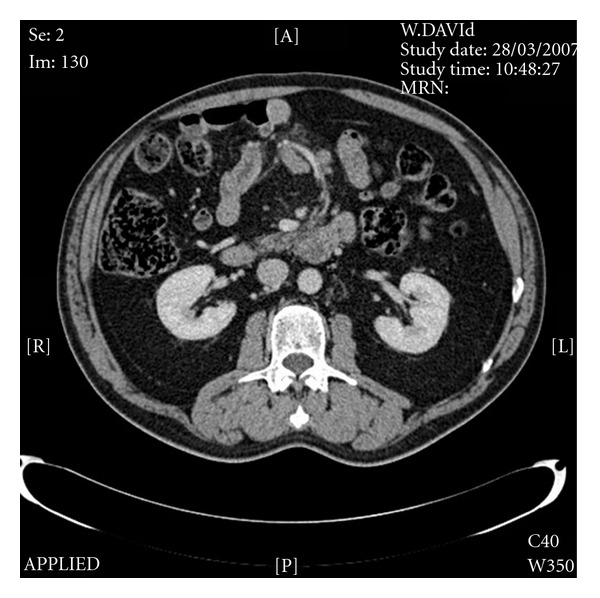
Axial reconstruction of abdominal computed tomography scan shows lymphadenopathy in the region of the superior mesenteric vein.
